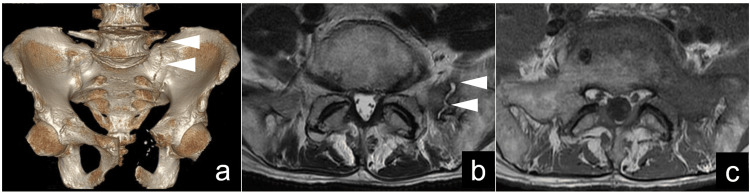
A fracture line in the left sacrum. (a) Three-dimensional computed tomography discloses a fracture line within the left sacrum. (b) T2-weighted magnetic resonance imaging demonstrates heightened signal intensity in the left sacrum. (c) T1-weighted magnetic Resonance imaging exhibits diminished signal intensity in the left sacrum.
